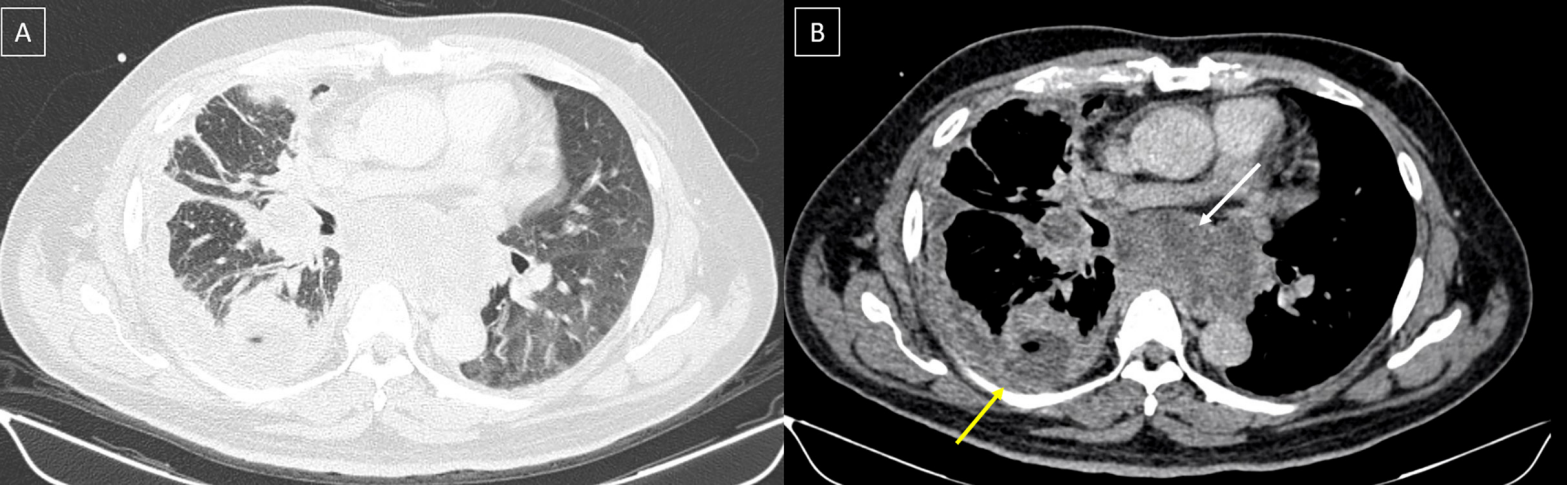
Imaging findings of the patient. Axial contrast‐enhanced CT of the thorax: Lung window (A) and soft‐tissue window (B) demonstrating a loculated right pleural effusion (yellow arrow) and mediastinal lymphadenopathy (white arrow).
